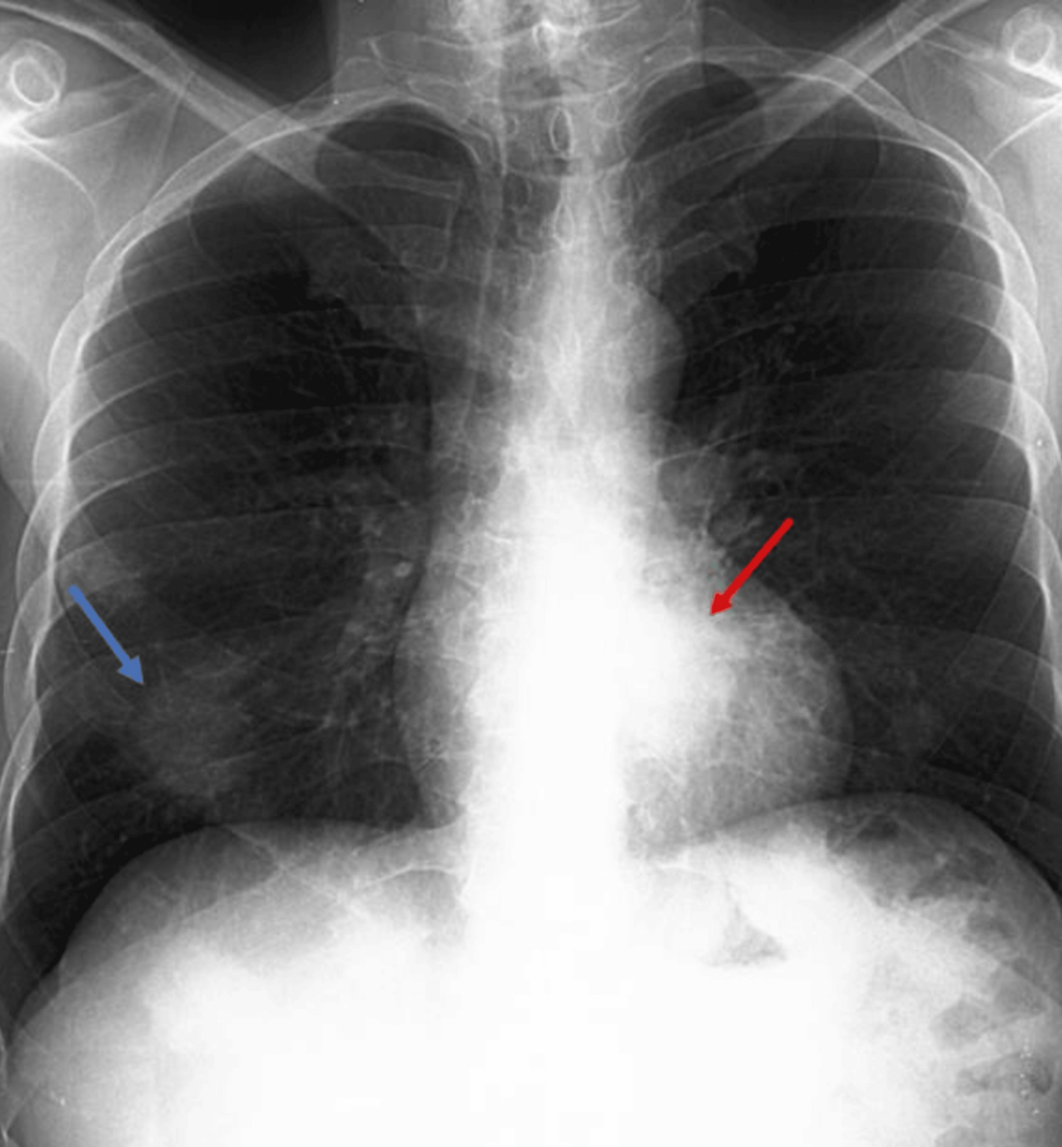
Chest X-ray The blue arrow indicates the right lower zone in a supradiaphragmatic position, which had the largest parenchymal opacity and measured 40 mm. The red arrow indicates a distinct oval opacity with sharp external borders and blurry internal margins that blended into the mediastinum, which was seen in the left lung base and retrocardiac on the same side.
